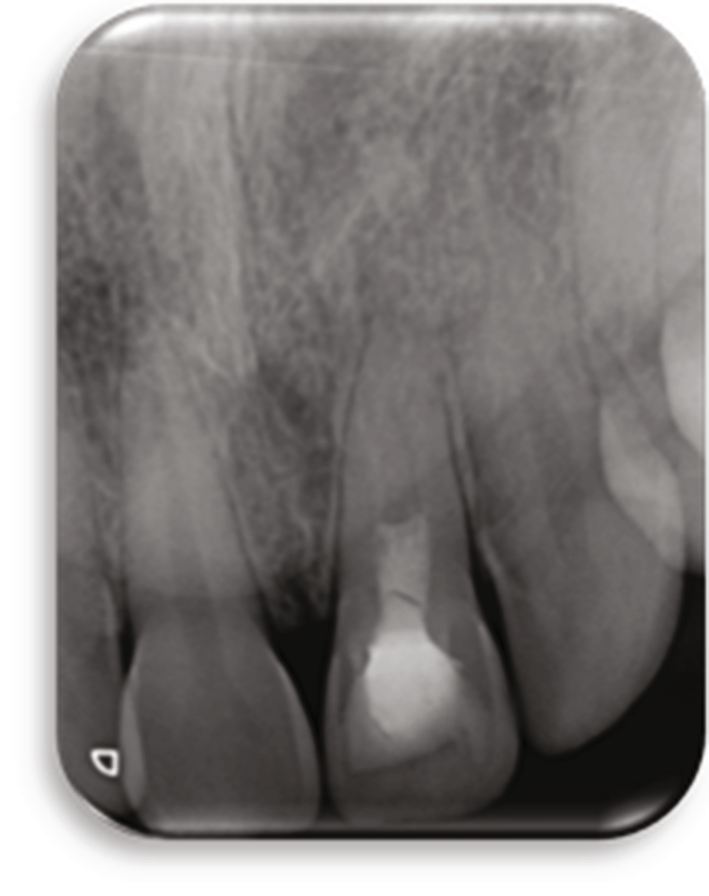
Preoperative radiograph: 7 years post‐RET radiograph showing the placement of MTA and considerable thickening of the dentinal walls and apical closure.
